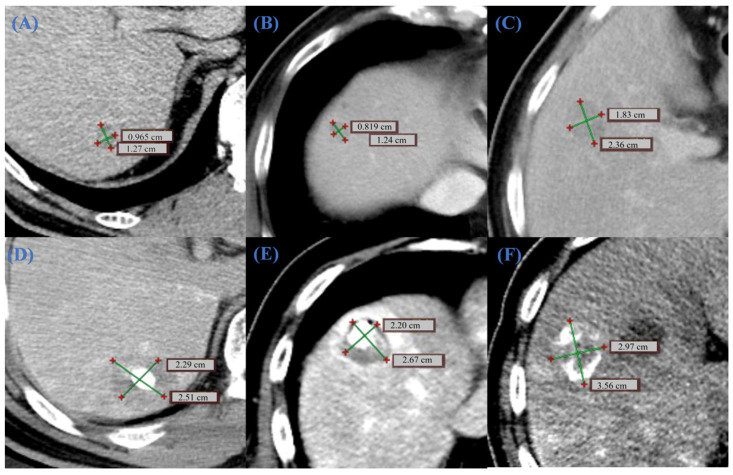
Comparison of tumor CT images before and after clinical surgical treatment. (A–C) shows the preoperative tumor size. (A) represents the CT image of patient 1, and (B,C) represent the CT images of different lesions in patient 2. (D–F) correspond to the immediate postoperative images at the aforementioned locations. The crosshairs in the images mark the long-axis and short-axis (cm) of the lesion and the postoperative coagulation zone. The comparison shows that the postoperative coagulation necrosis zone significantly exceeds the original tumor boundary, and both its long and short axis measurements are greater than the preoperative tumor morphology, confirming that the coagulation zone completely and effectively covered the target tumor. The green crosshairs indicate the measured axes of the lesion or coagulation zone, and the red markers denote the endpoints of the measurements.
